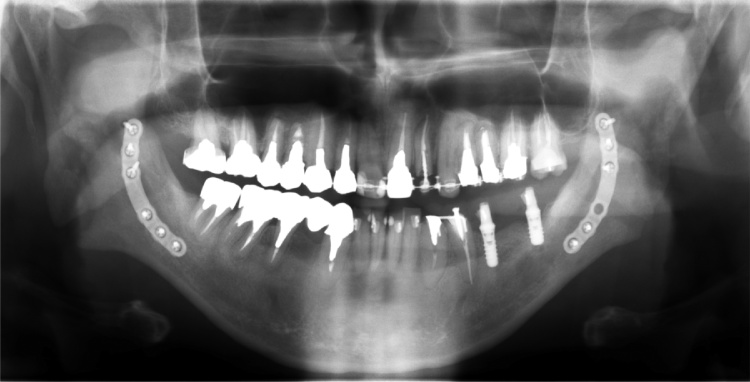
Panoramic X-ray at 3 months after the surgery in Case 1.
